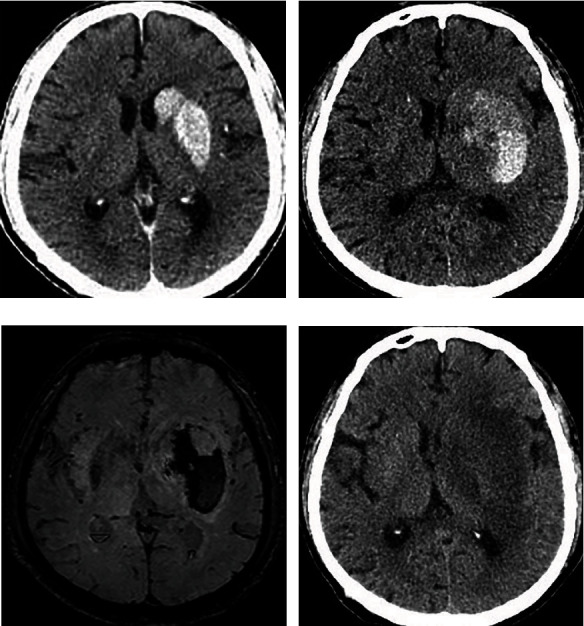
CT scan of a 62-year-old man who had a sudden onset of impaired right limb movement. (a). The non-enhanced brain CT scan obtained immediately after intra-arterial thrombolysis showing a hyperdense area in the head portion of the left caudate nucleus and the entire left lentiform nucleus. Representative type II, namely solid “mass” shaped lesions with well-defined boundaries and without mass effect. (b). Follow-up CT scan obtained 48 hours after the end of intra-arterial thrombolysis. The range of the hyperdense area increased slightly and the attenuation decreased. The patient was not accompanied by deterioration of neurological symptoms. (c). On the 2nd day after the procedure, susceptibility weighted imaging showed patchy low signal in the left basal ganglia region, indicating hematoma formation. (d). Two weeks after the procedure, the left basal ganglia hematoma had been completely absorbed.
